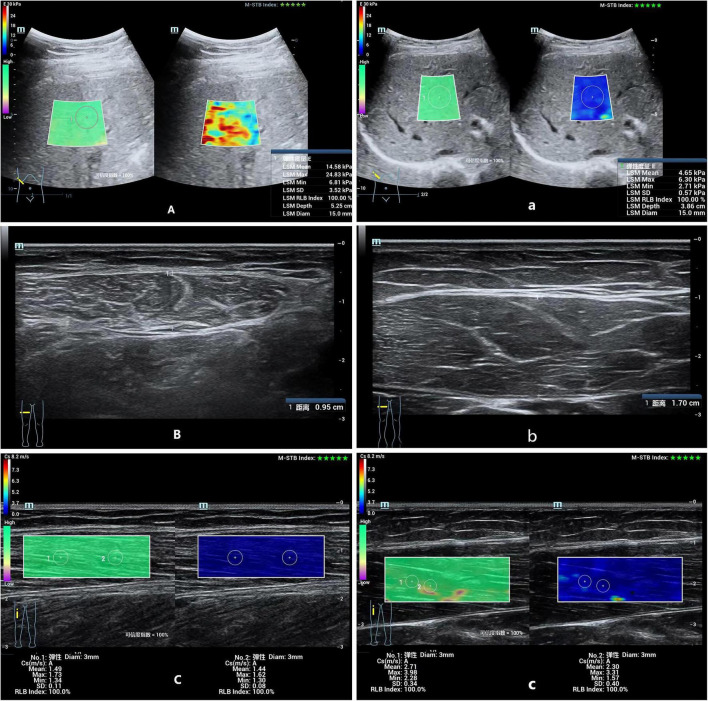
Comparison of selected ultrasound parameters between patients with CRS and healthy individuals. Capital letters denote patients with CRS. Lowercase letters denote healthy individuals. (A) Liver elastic modulus, (B) rectus femoris thickness, (C) rectus femoris shear wave velocity. (a) Liver elastic modulus, (b) rectus femoris thickness, (c) rectus femoris shear wave velocity. CRS, Cirrhosis-Related Sarcopenia.
